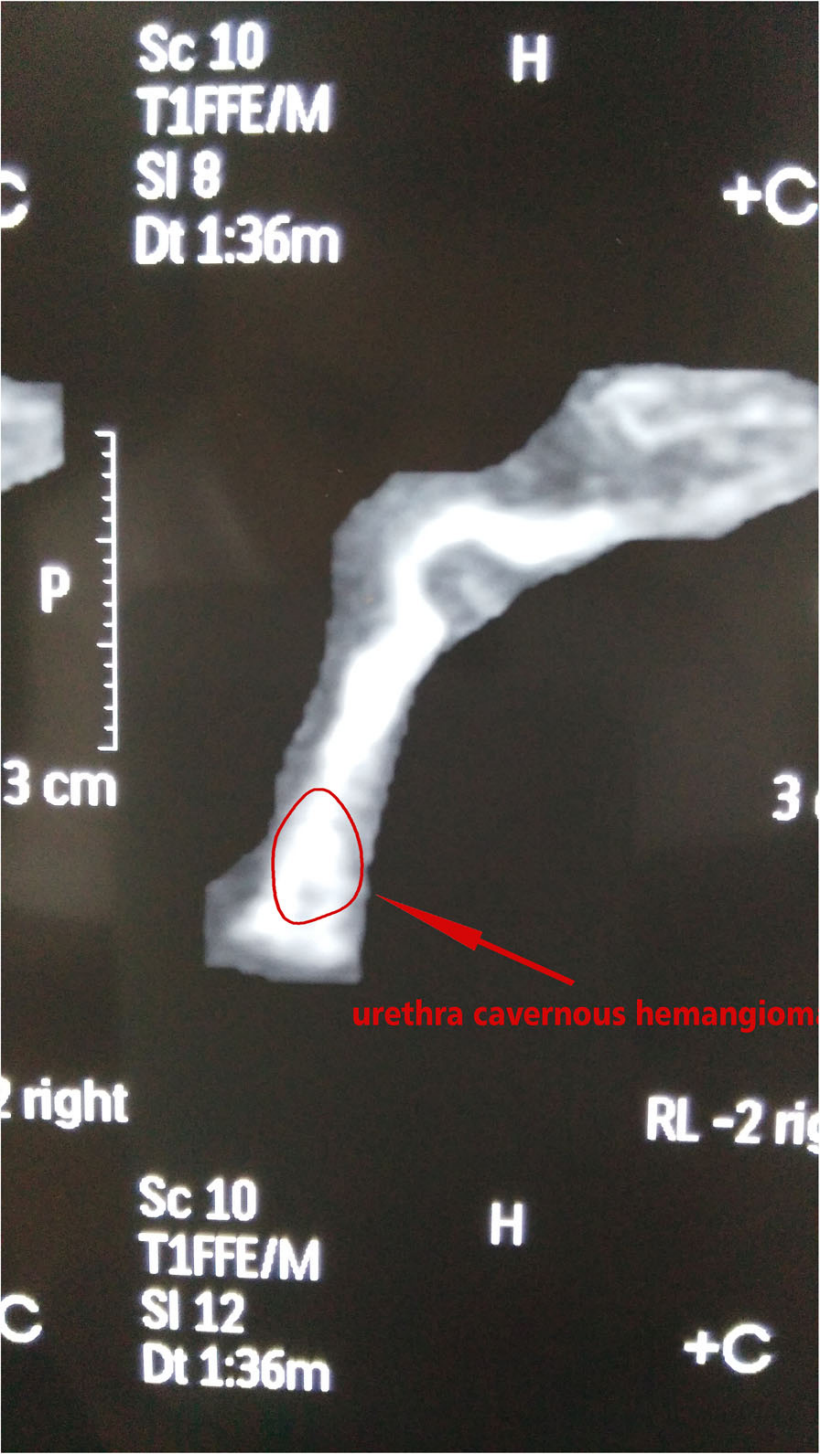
Preoperative penis MRI reconstruction shows a high signal at the front of the penis
